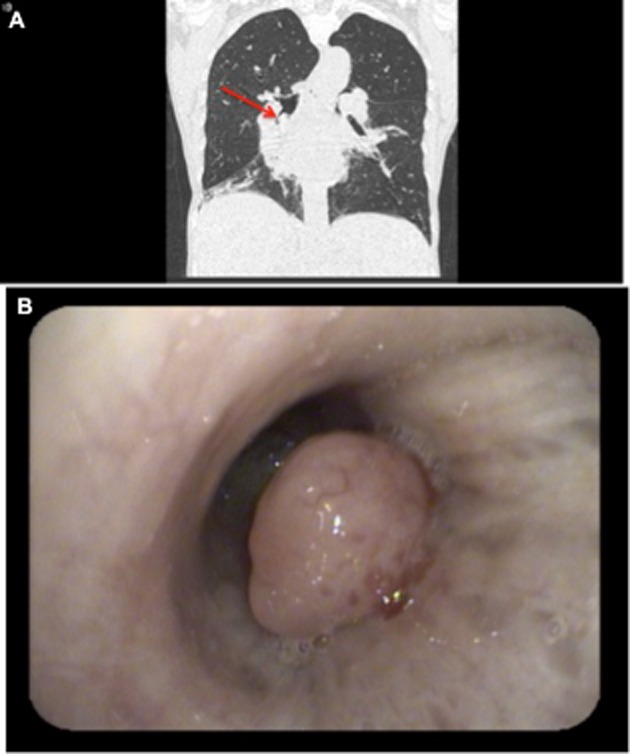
(A) Computed tomography image showing multiple nodular lesions causing varying degrees of obstruction in the central airways. Note the obstructing polyp in the bronchus intermedius (arrow). (B) A chronic lymphocytic leukemia nodular lesion causing obstruction of the bronchus intermedius lumen.
